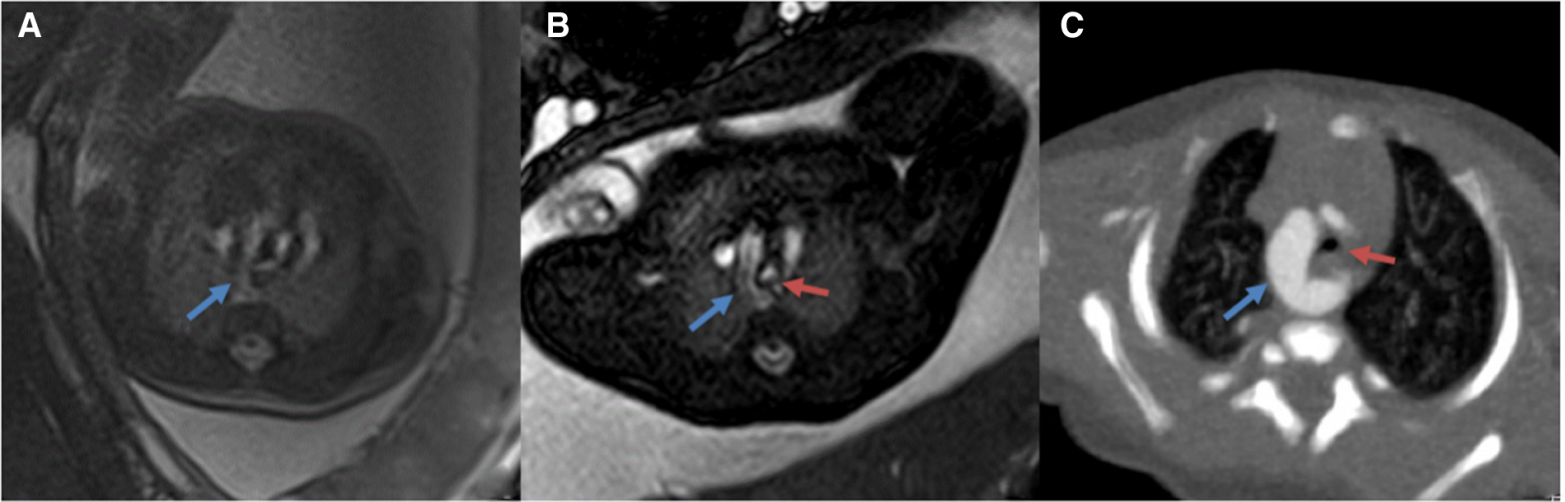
(A,B) comparison of 3.0 T(A) vs. corresponding 1.5 T (B) MR images of the fetal great vessels showing the advantages of increased resolution at 1.5 T (C): Postnatal CTA images (12 days) blue arrows show vascular ring; red arrows show the trachea.
